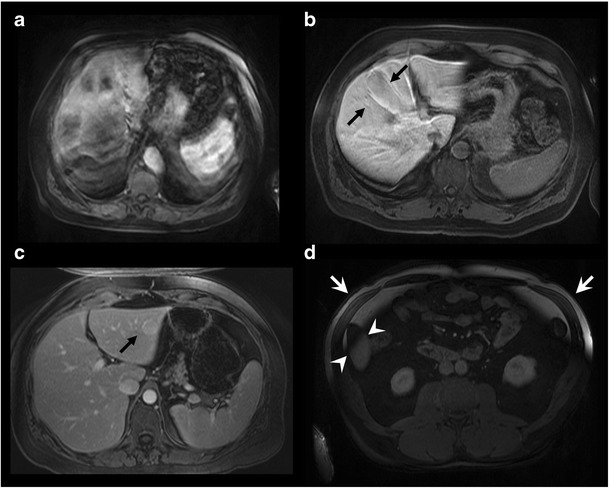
Imaging pitfalls which may degrade liver segmentation on MRI. Axial T1-weighted fat-saturated images with contrast injection depict the following artefacts: a Severe motion artefact. b Partial volume averaging of the liver parenchyma with the gallbladder (*arrows*). c Ghost artefact with the aorta (*arrow*). d Inhomogeneous fat saturation (*white arrows*) and fat-water swap in the liver (*arrowheads*) [13]
